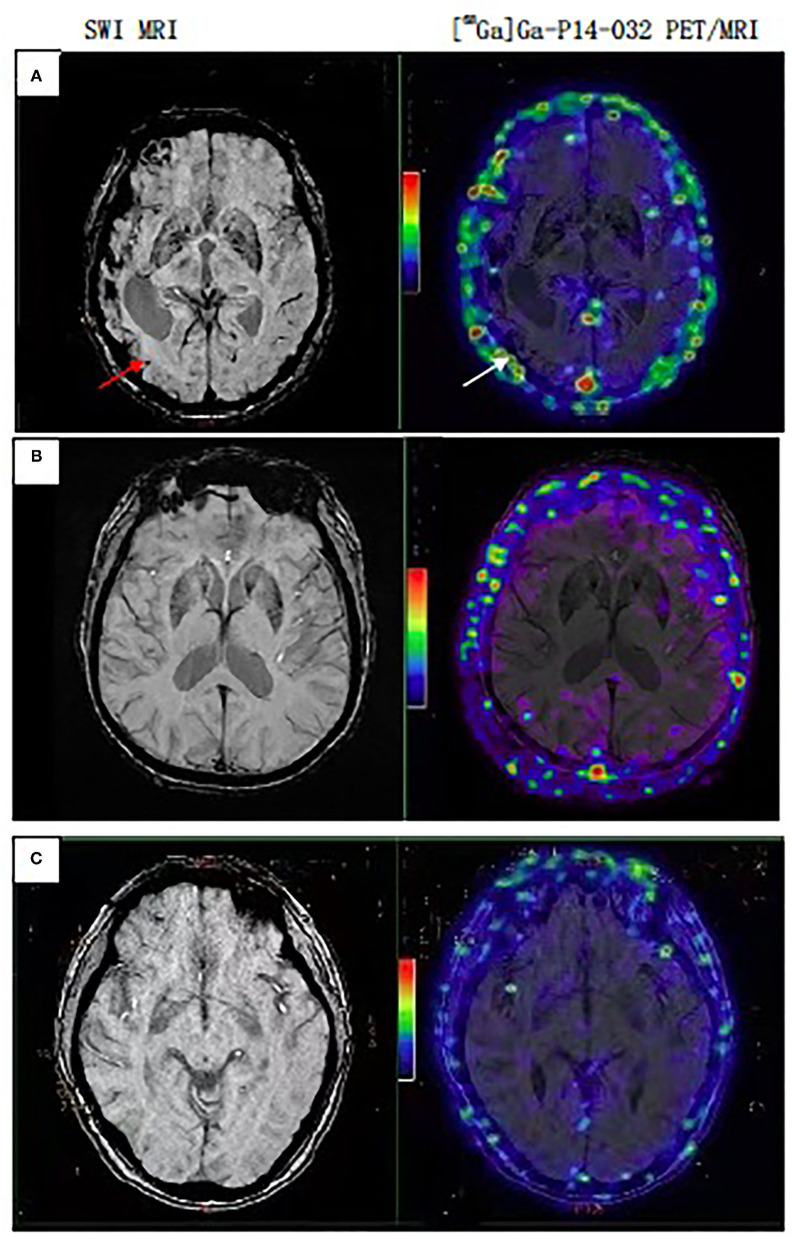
PET/MRI scan sets representative of CAA, AD, Normal subjects. (A) Case 7, female, 70 years, probable CAA patient, multiple CMBs on MRI, white arrow point to the same point to the same area with [^68^Ga]Ga-p14-032 retention on PET/MRI. (B) Case 5, male, 84 years, AD patient. (C) Case 3, female, 57 years, cognitive normal subject. Cases (B,C) show only non-specific binding in extracerebral tissues.
